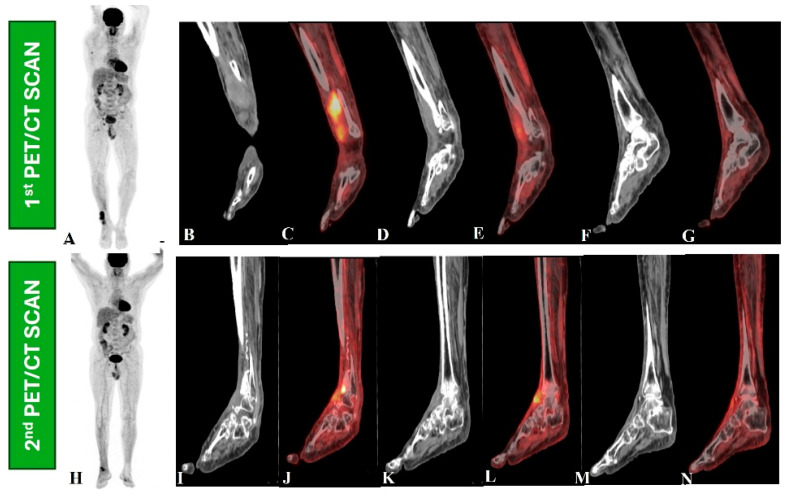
A 66-year-old male had wide-excision surgery for recurrent soft tissue sarcoma in the right leg. Following surgery, the CT component in the first PET/CT scan (upper row, B–G) revealed a mass lesion along the anterior aspect of the right distal tibia (B,D,F) and increased [^18^F]-FDG uptake on the maximum intensity projection (MIP) PET image (A) and fused PET and CT images (C,F), indicating active residual disease. The patient then received radiation therapy and returned for a response assessment PET/CT scan (lower row, I–N), which revealed ill-defined soft tissue thickening along the anterior aspect of the right distal tibia in a CT scan (I,K,M) and metabolically active cortical erosion with reduced [^18^F]-FDG avidity, suggesting a partial metabolic response (H,J,L,N).
